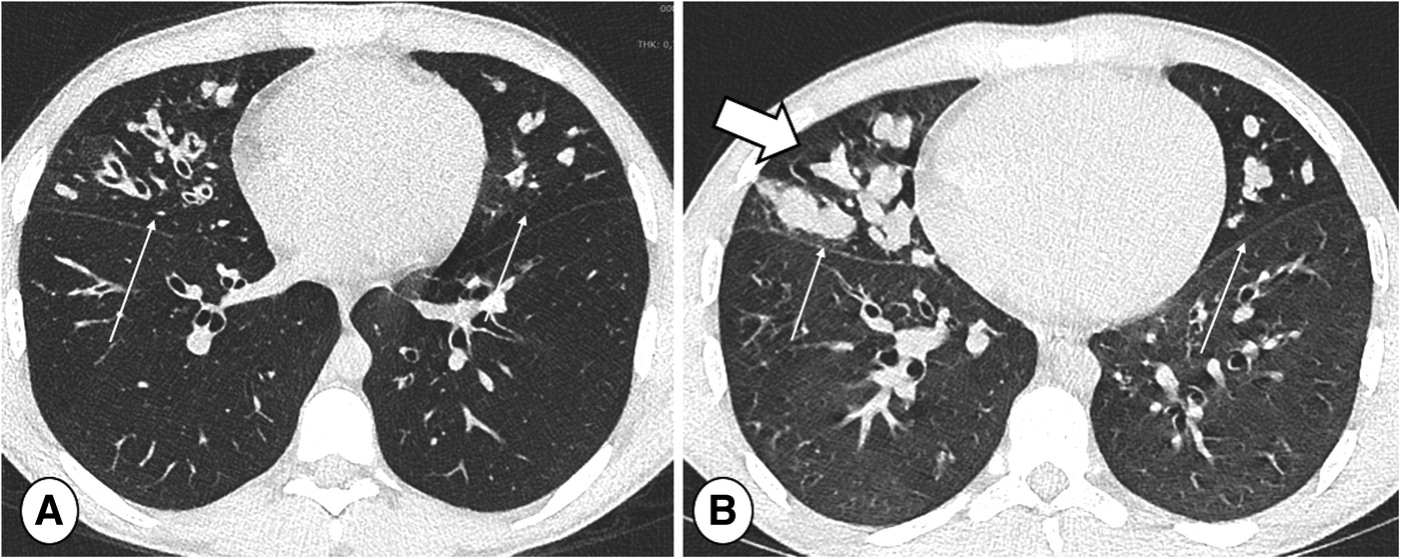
End-inspiratory CT of a cystic fibrosis patient (A) without and (B) with respiratory tract exacerbation, lung window. Note bronchiectasis and mucus plugs, especially in the middle lobe and lingula (thin arrows). The only difference indicating RTE is an increase in central mucus plugs (thick arrow).
